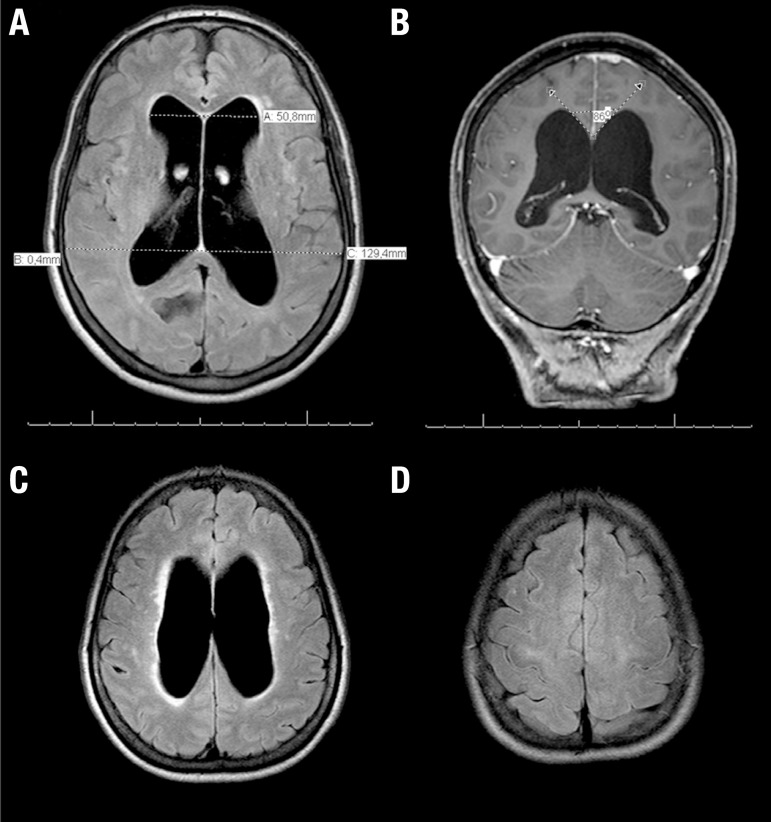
Neuroimaging in NPH (A) Axial FLAIR MRI
